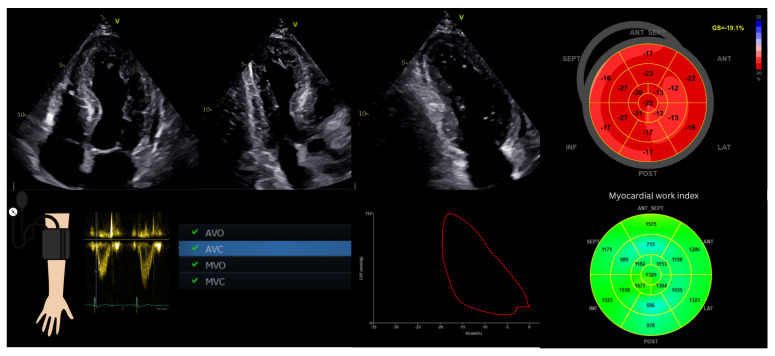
Myocardial work assessment includes two-dimensional grayscale imaging in apical two-, three-, and four-chamber views, followed by global longitudinal strain and left ventricular bull’s-eye analysis. Activation of the myocardial work module allows input of brachial blood pressure and determination of valvular timing. The output includes regional and global myocardial work indices, pressure–strain loops, and derived parameters such as global constructive and wasted work. ANT_SEPT, antero-septal; ANT, anterior; LAT, lateral; POST, posterior; INF, inferior; SEPT, septal; GS, global strain; AVO, aortic valve opening; AVC, aortic valve closure; MVO, mitral valve opening; MVC, mitral valve closure; V, apical view orientation marker.
